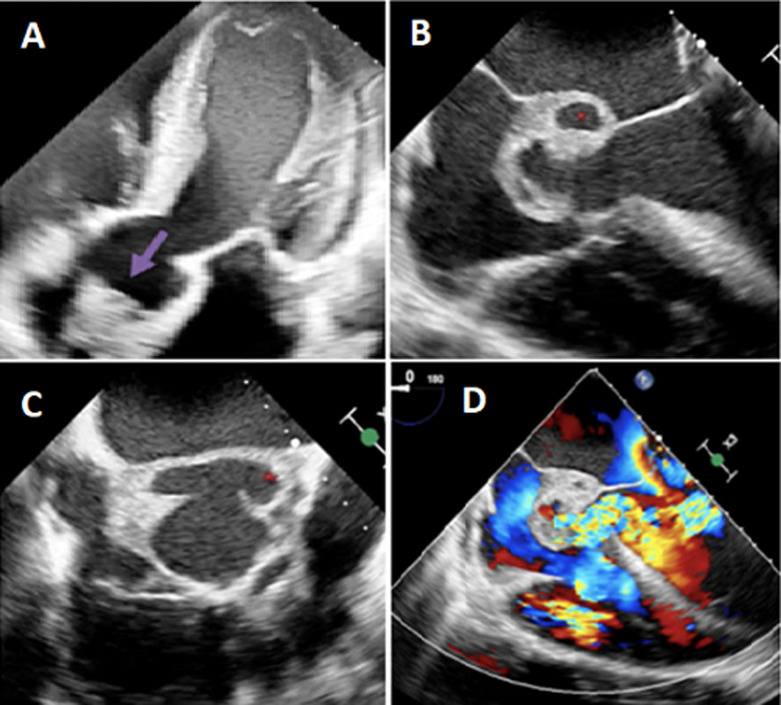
clinical case 1; A) transthoracic echocardiography five chamber view showing a vegetation in the aortic valve (arrow); (B,C,D) transesophageal echocardiography showing abscess in aortic root (star) with Doppler signal showing aorta regurgitation
